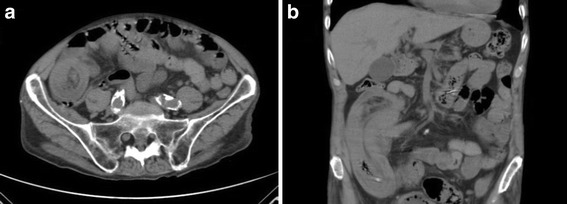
Abdominal computed tomography showed the characteristic inhomogeneous “target-shaped” soft tissue mass with a layering effect in the axial plane (a) and the typical “sausage-shaped” soft tissue mass in the coronal plane (b)
